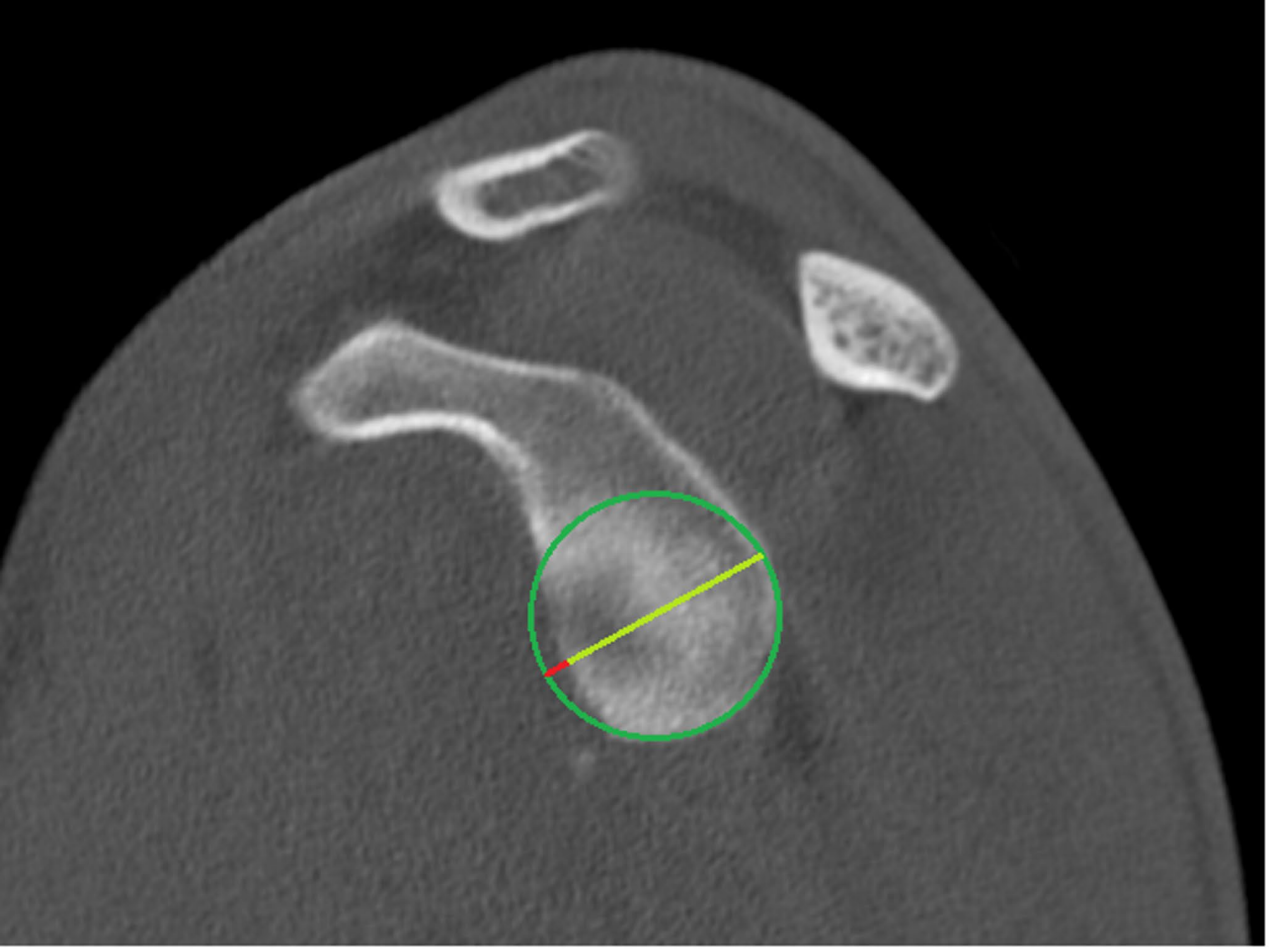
Measurement of the glenoid track using computed tomography as an example. Measurement of the defect length d (red line) and measurement of the diameter of the glenoid D (red line and light green line)
